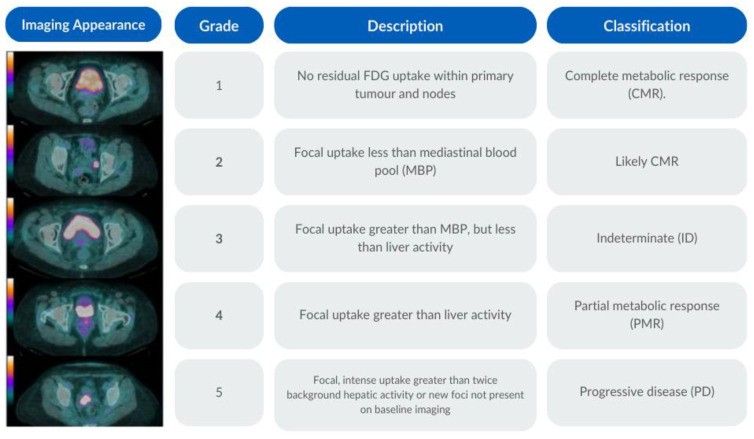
2-[^18^F]FDG PET-CT response assessment scale.
